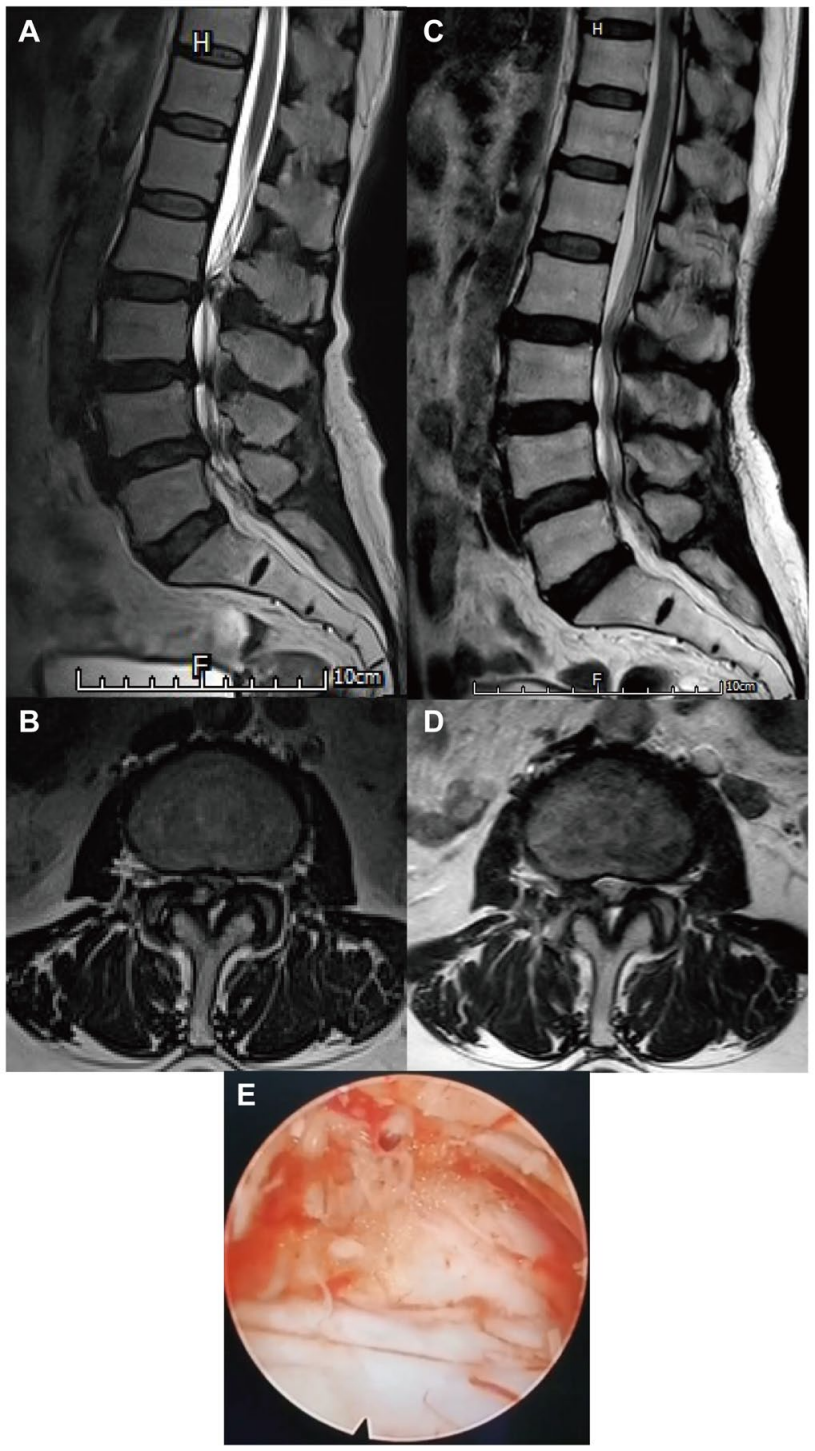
Radiological images of a patient with lumbar facet joint cyst (FJC) who underwent uniportal full-endoscopic (UFE) surgery through a transforaminal approach. (A,B) Preoperative magnetic resonance imaging (MRI) of the lumbar spine revealed one cyst located at the right facet joint at the L2-3 level. (C,D) A postoperative MRI of the lumbar spine showed that the FJC was completely removed. (E) Endoscopic view of the FJC during the UFE surgery.
